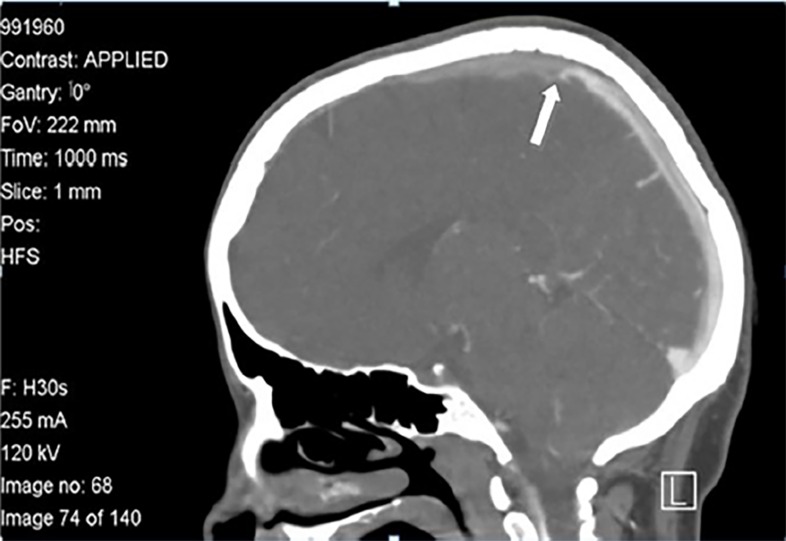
Sagittal computed tomography venogram of the brain showing filling defects involving most of the superior sagittal sinus (arrow), except the posterior portion, suggesting superior sagittal sinus thrombosis.
